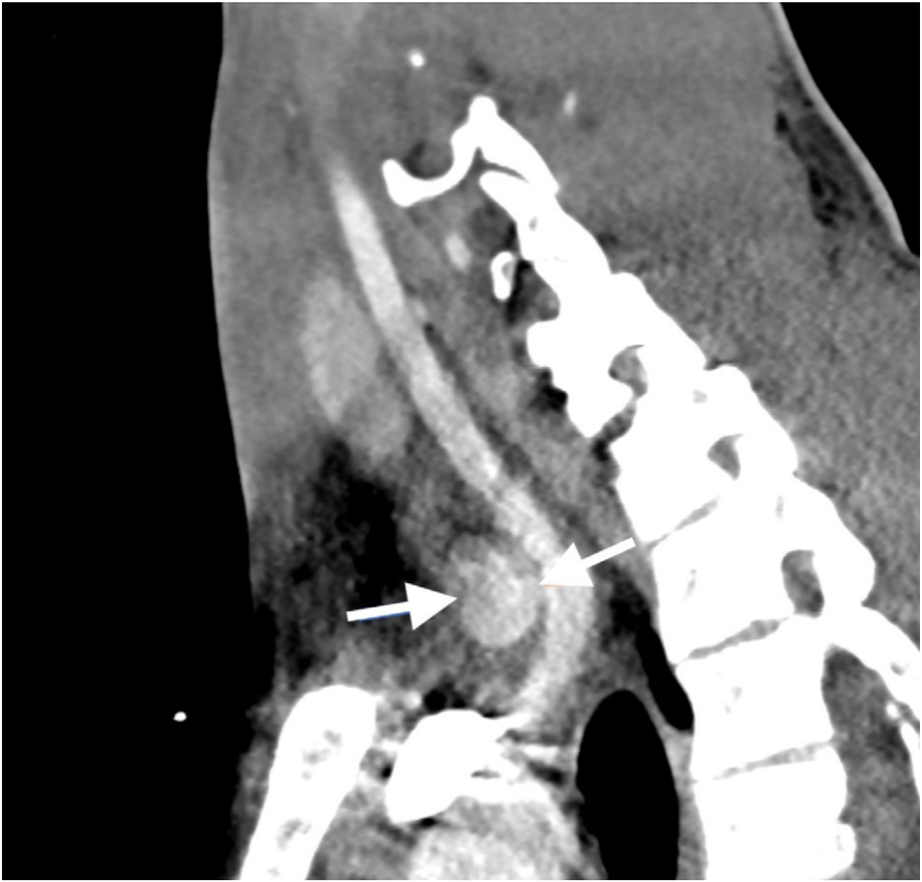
Sagittal multiplanar reconstruction of the computed tomography angiogram of the head and neck demonstrates a 1.8 cm amorphous, hyperdense, oval structure abutting the anterior aspect of the junction of the brachiocephalic and right common carotid arteries, with an apparent connecting neck extending from the brachiocephalic artery (arrows), suggesting a pseudoaneurysm or contained rupture.
